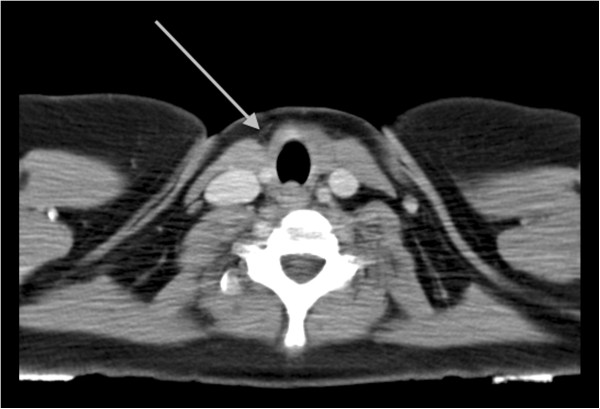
Computed tomography of the neck region, one year after therapy (surgery and chemotherapy); no local recurrence or regional metastases.
